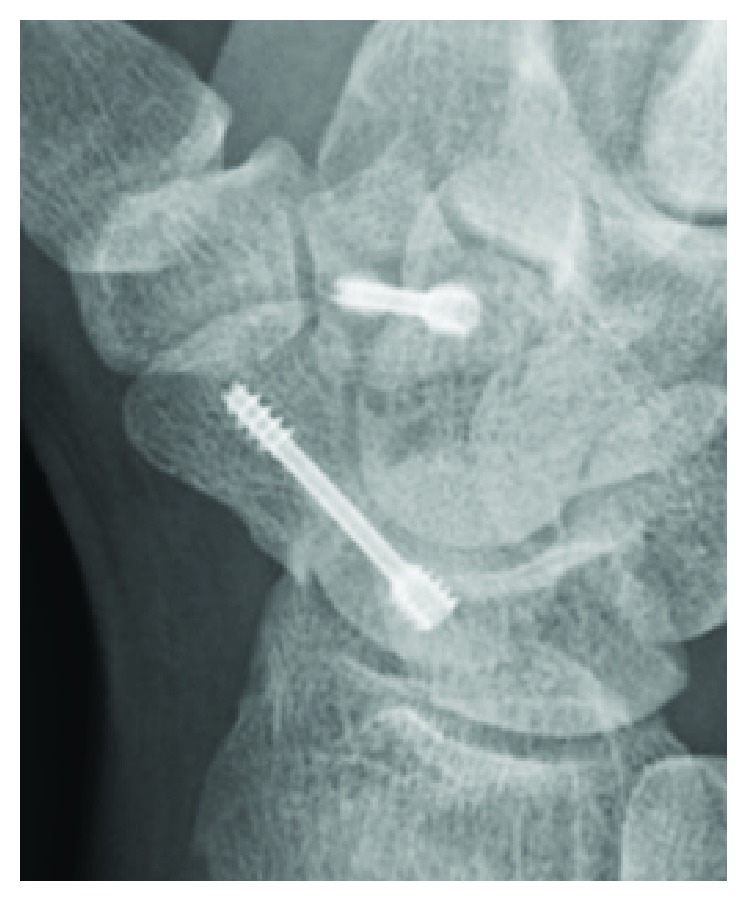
X-ray films at the 5-month follow-up visit. The fracture line was still visible in the scaphoid, but most part of the fracture site was united.
